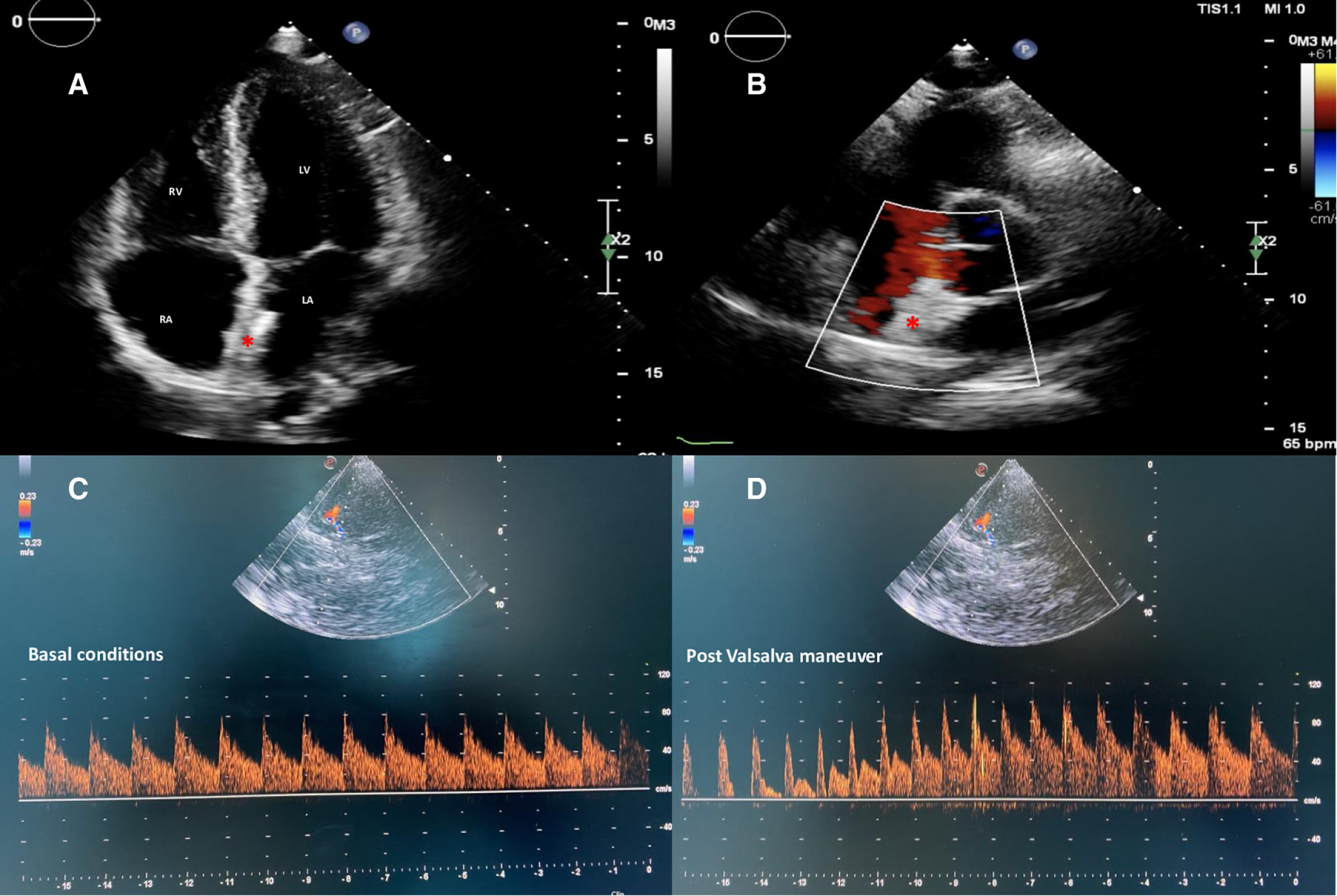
Two-dimensional TTE color Doppler in apical four-chambers (A) and parasternal short-axis (B) views showing a correct and stable position of the equally sized discs nitinol device (red asterisk) without residual shunt. cTCD confirming abolition of the interatrial shunt both in basal conditions (C) and after adequate Valsalva maneuver (D). TTE, transthoracic echocardiography; cTCD, contrast transcranial Doppler.
